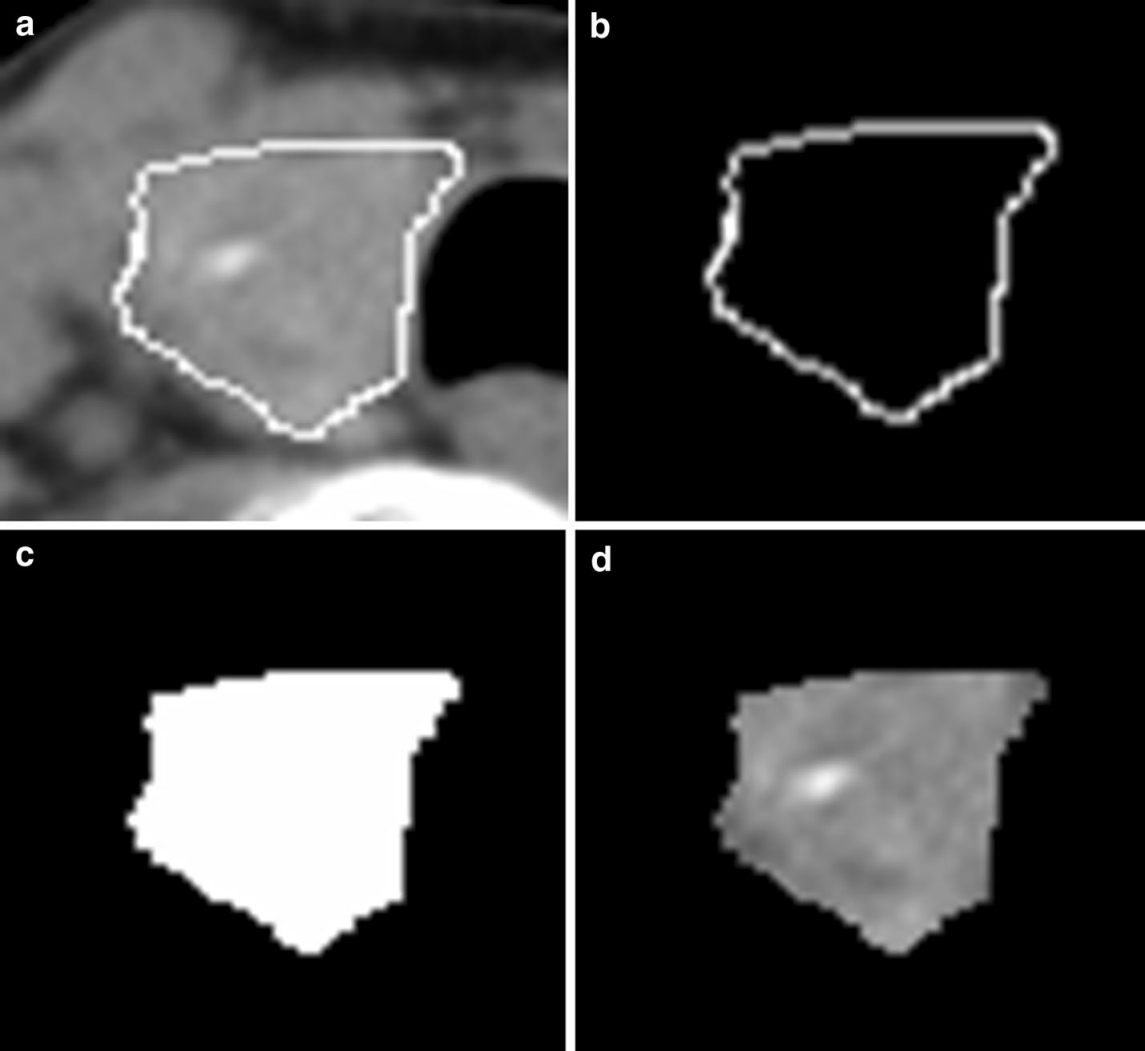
The procedure of ROI extraction in thyroid CT image. a The contour of a thyroid with malignant nodule. b Binary contour. c Mask of thyroid tissue. d Target ROI of thyroid tissue
